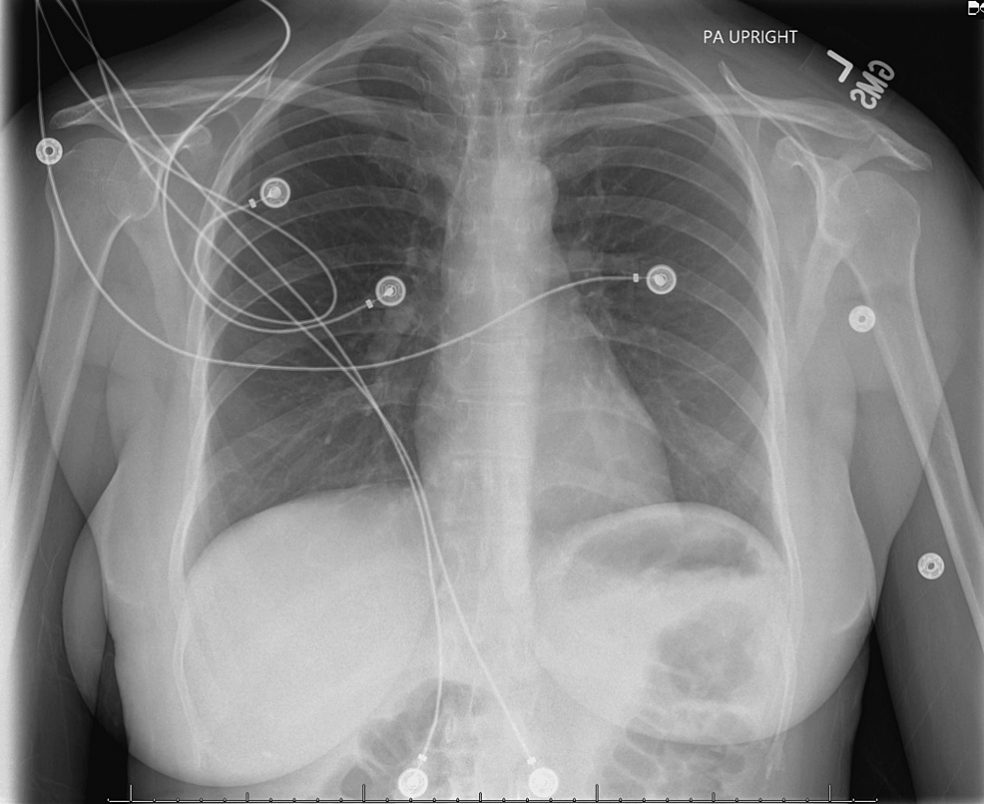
A single-view chest radiograph that was obtained in the emergency department.
